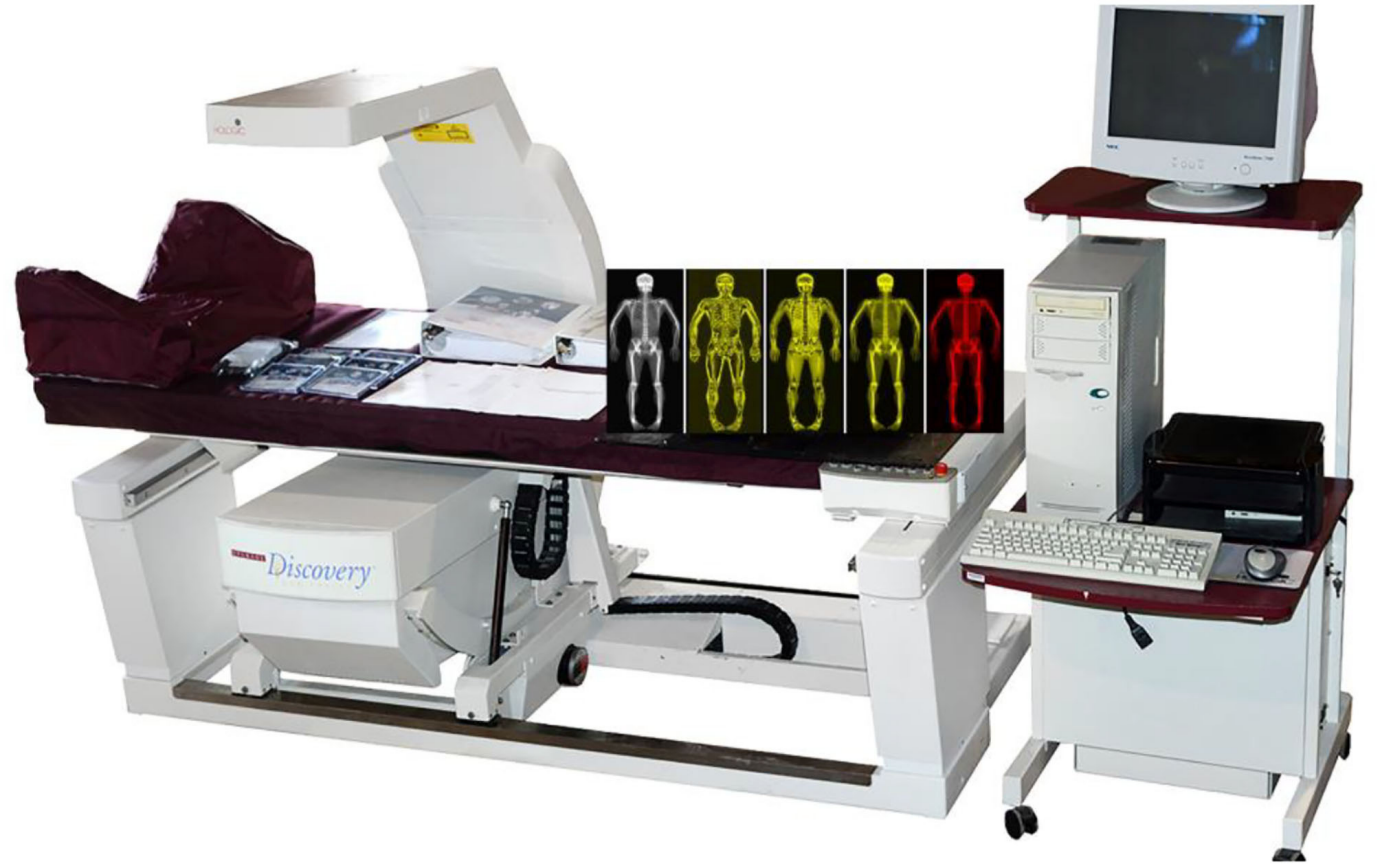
A dual-energy X-ray absorptiometry (DXA) scanner (Hologic QDR-4500A).
